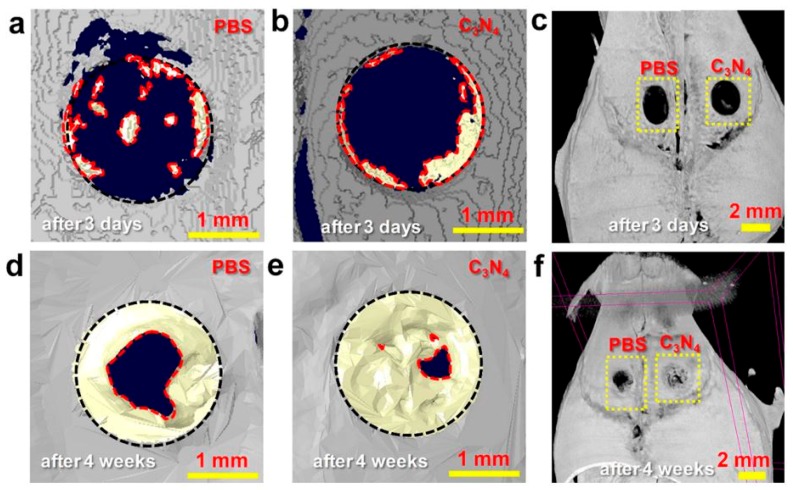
(a–f) Three-dimensional μ-CT images after 3 days or 4 weeks of PBS and C_3_N_4_ nanosheet-assisted treatment for the enhanced repair of cranial bone defect under red light in vivo [88]. C_3_N_4_ sheets showed the potential for promoting bone formation. Reproduced from [88] with permission from American Chemical Society, 2018.
